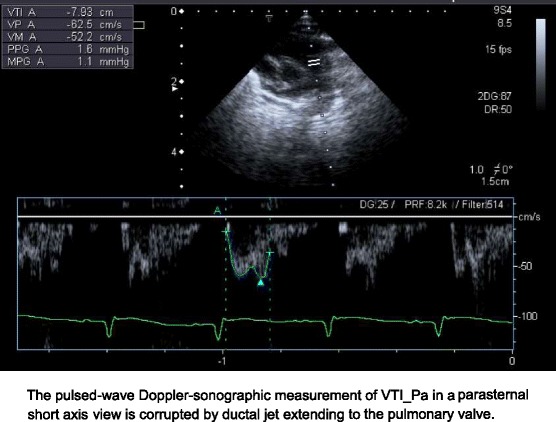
Measurement of VTI_Pa in a parasternal short axis view. The pulsed-wave Doppler-sonographic measurement of VTI_Pa in a parasternal short axis view is corrupted by ductal jet extending to the pulmonary valve
